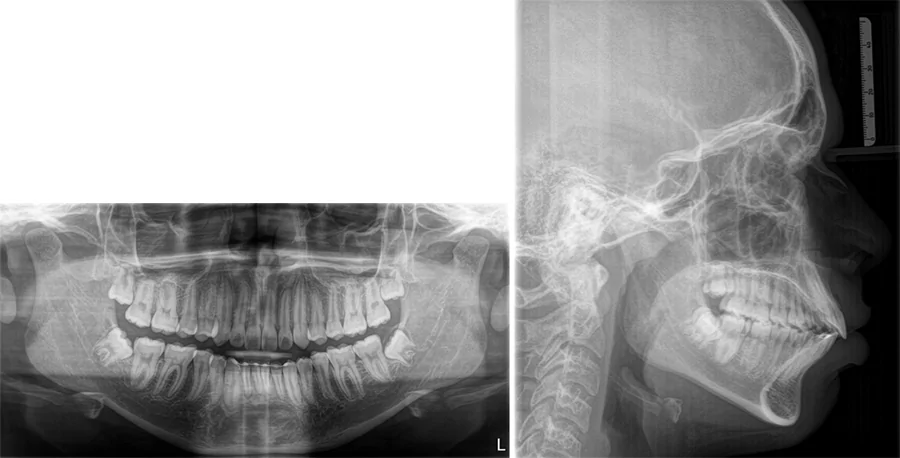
Control radiographs at the end of treatment
